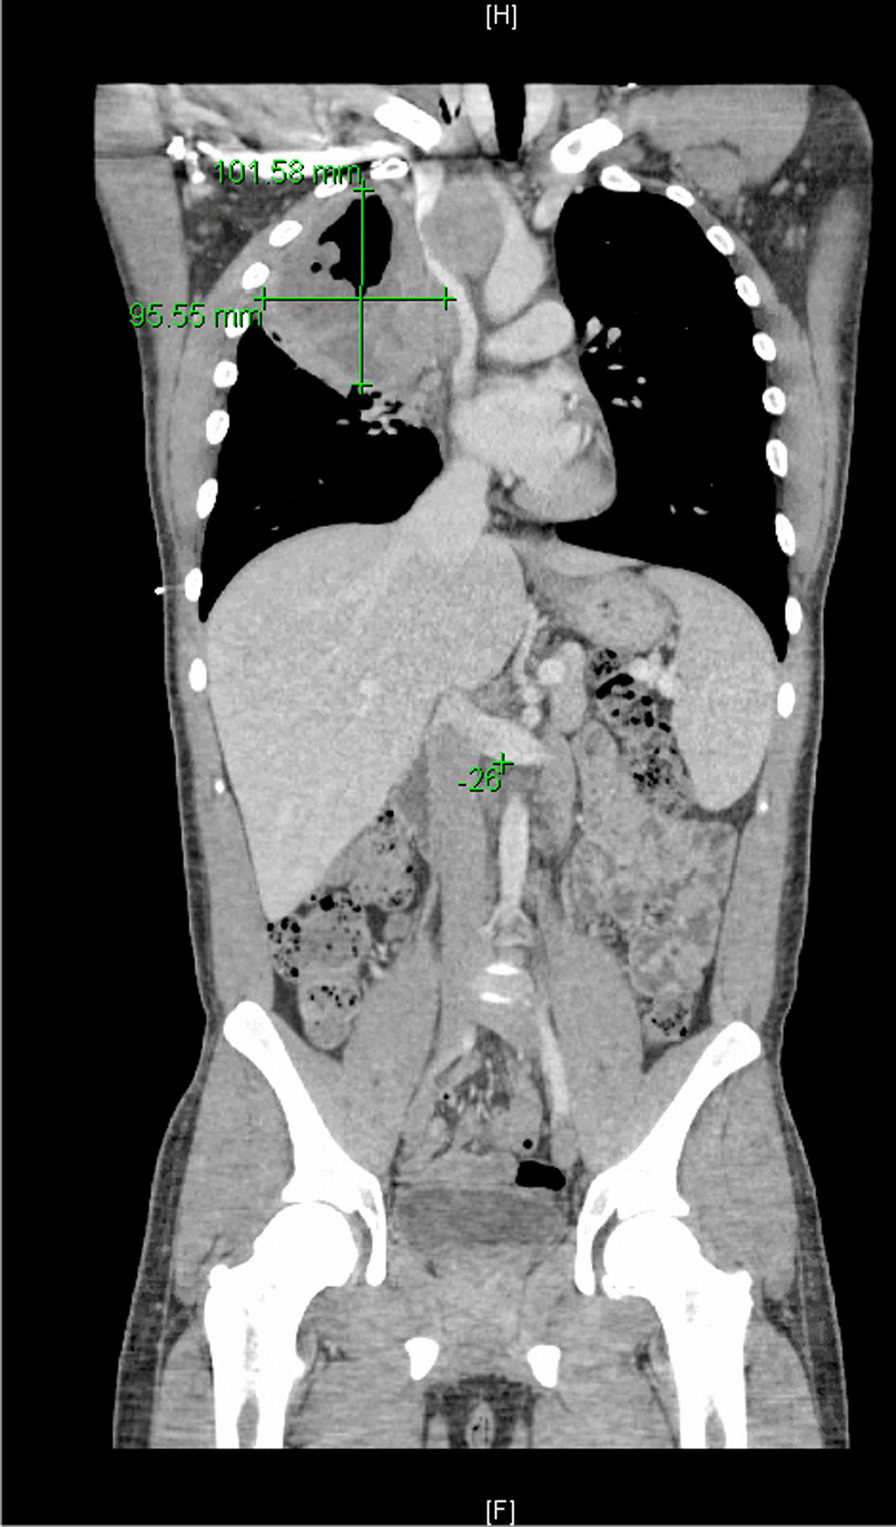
Follow-up CT chest/abdomen/pelvis (coronal image). Enlarging right upper lobe cavitary lesion
